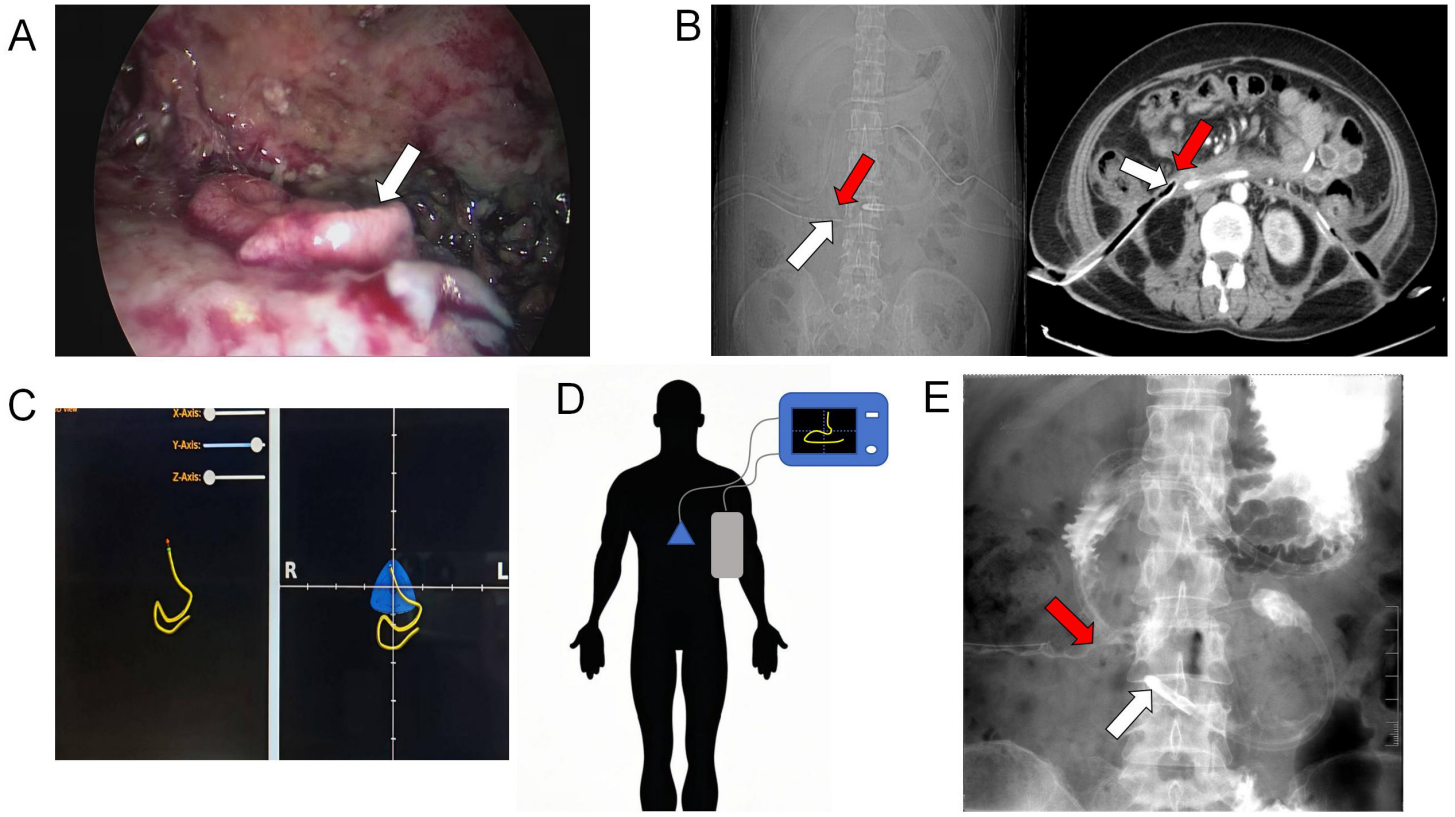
(A) Intraoperative visualization of the duodenal fistula orifice (white arrow); (B) Post-operative CT demonstrated the position of the drainage tube tip (white arrow) and the location of the duodenal fistula (red arrow); (C, D) use of electromagnetic navigation for inserting the nasojejunal tube; (E) the jejunal feeding tube tip position (white arrow) and duodenal fistula orifice localization (red arrow) was achieved via water-soluble iodinated contrast radiography.
